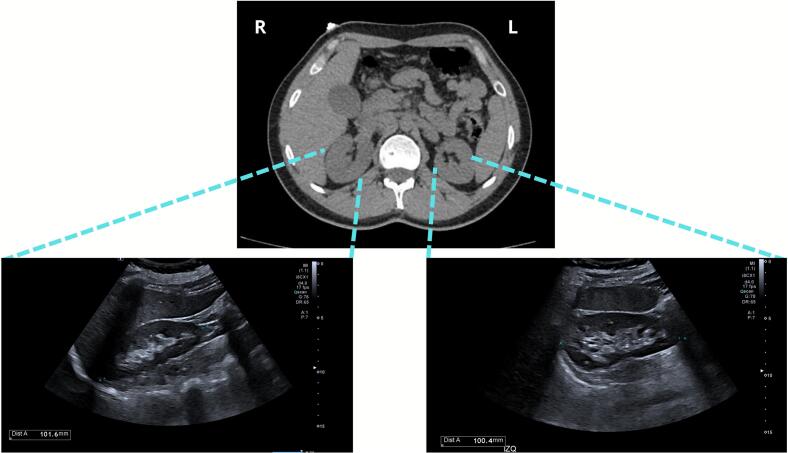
Renal imaging during evaluation for suspected tubulopathy. Abdominal computed tomography (CT) and corresponding renal ultrasound images of the right and left kidneys. Imaging demonstrated normal renal morphology and size (approximately 10 cm longitudinal length), with no evidence of structural abnormalities such as stones, masses, or pelvicalyceal dilation. These findings support the diagnosis of a functional renal tubulopathy in the absence of anatomical defects.
